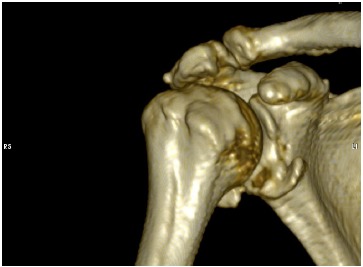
A three-dimensional reconstruction CT-image demonstrating a bony-Bankart lesion.
